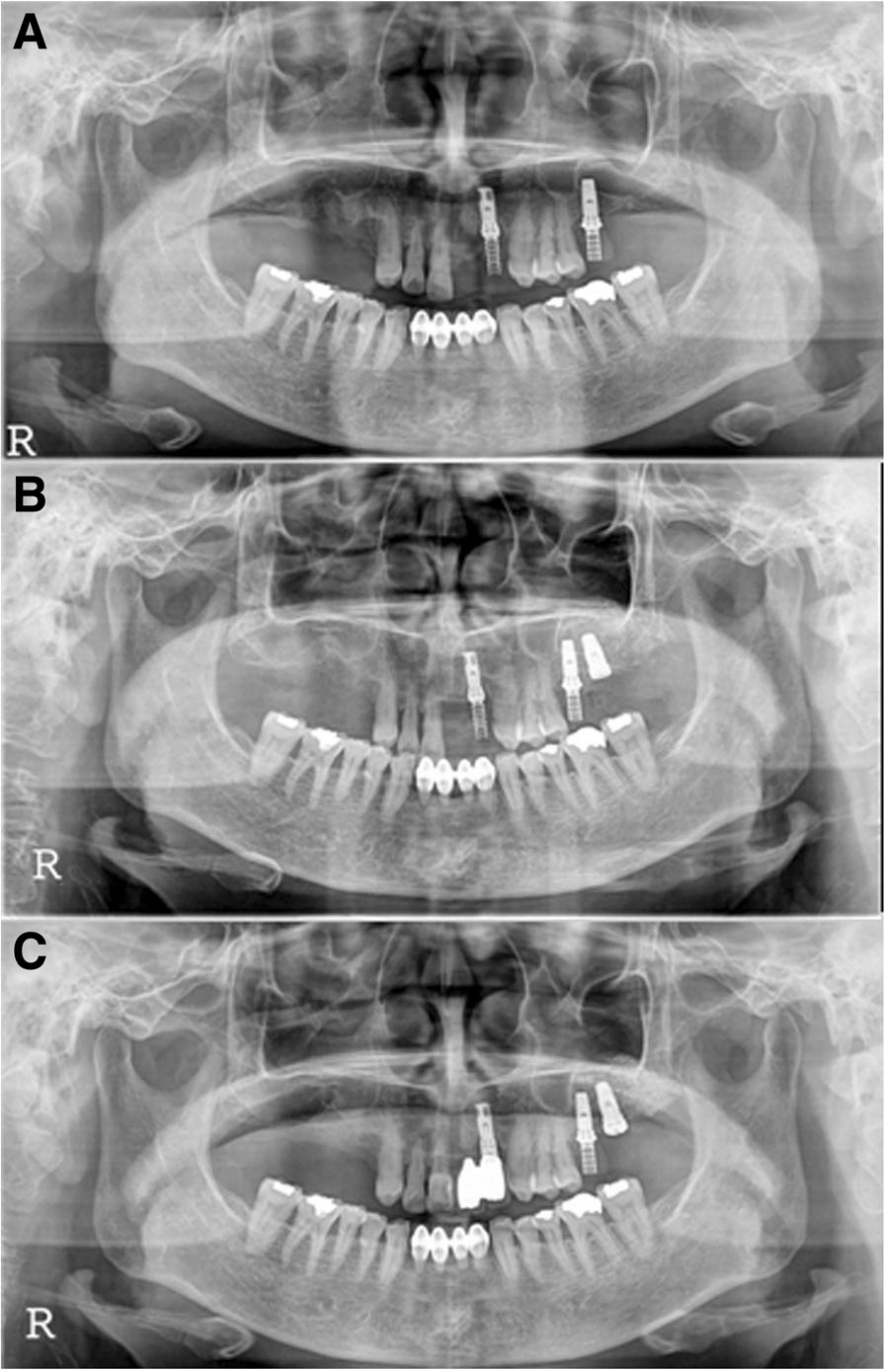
F2, Panoramic radiograph, #27i surgery a initial panoramic radiograph, b postoperative panoramic radiograph, c #27i second operation
